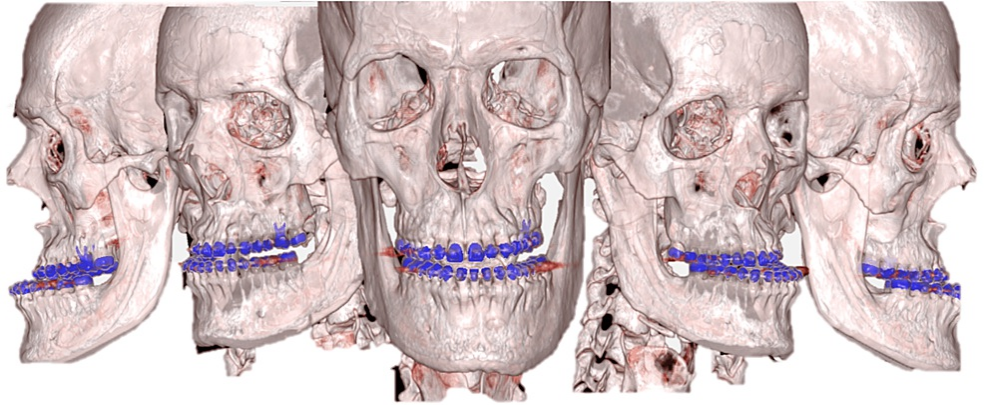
Initial CT scan with tridimensional reconstruction.
